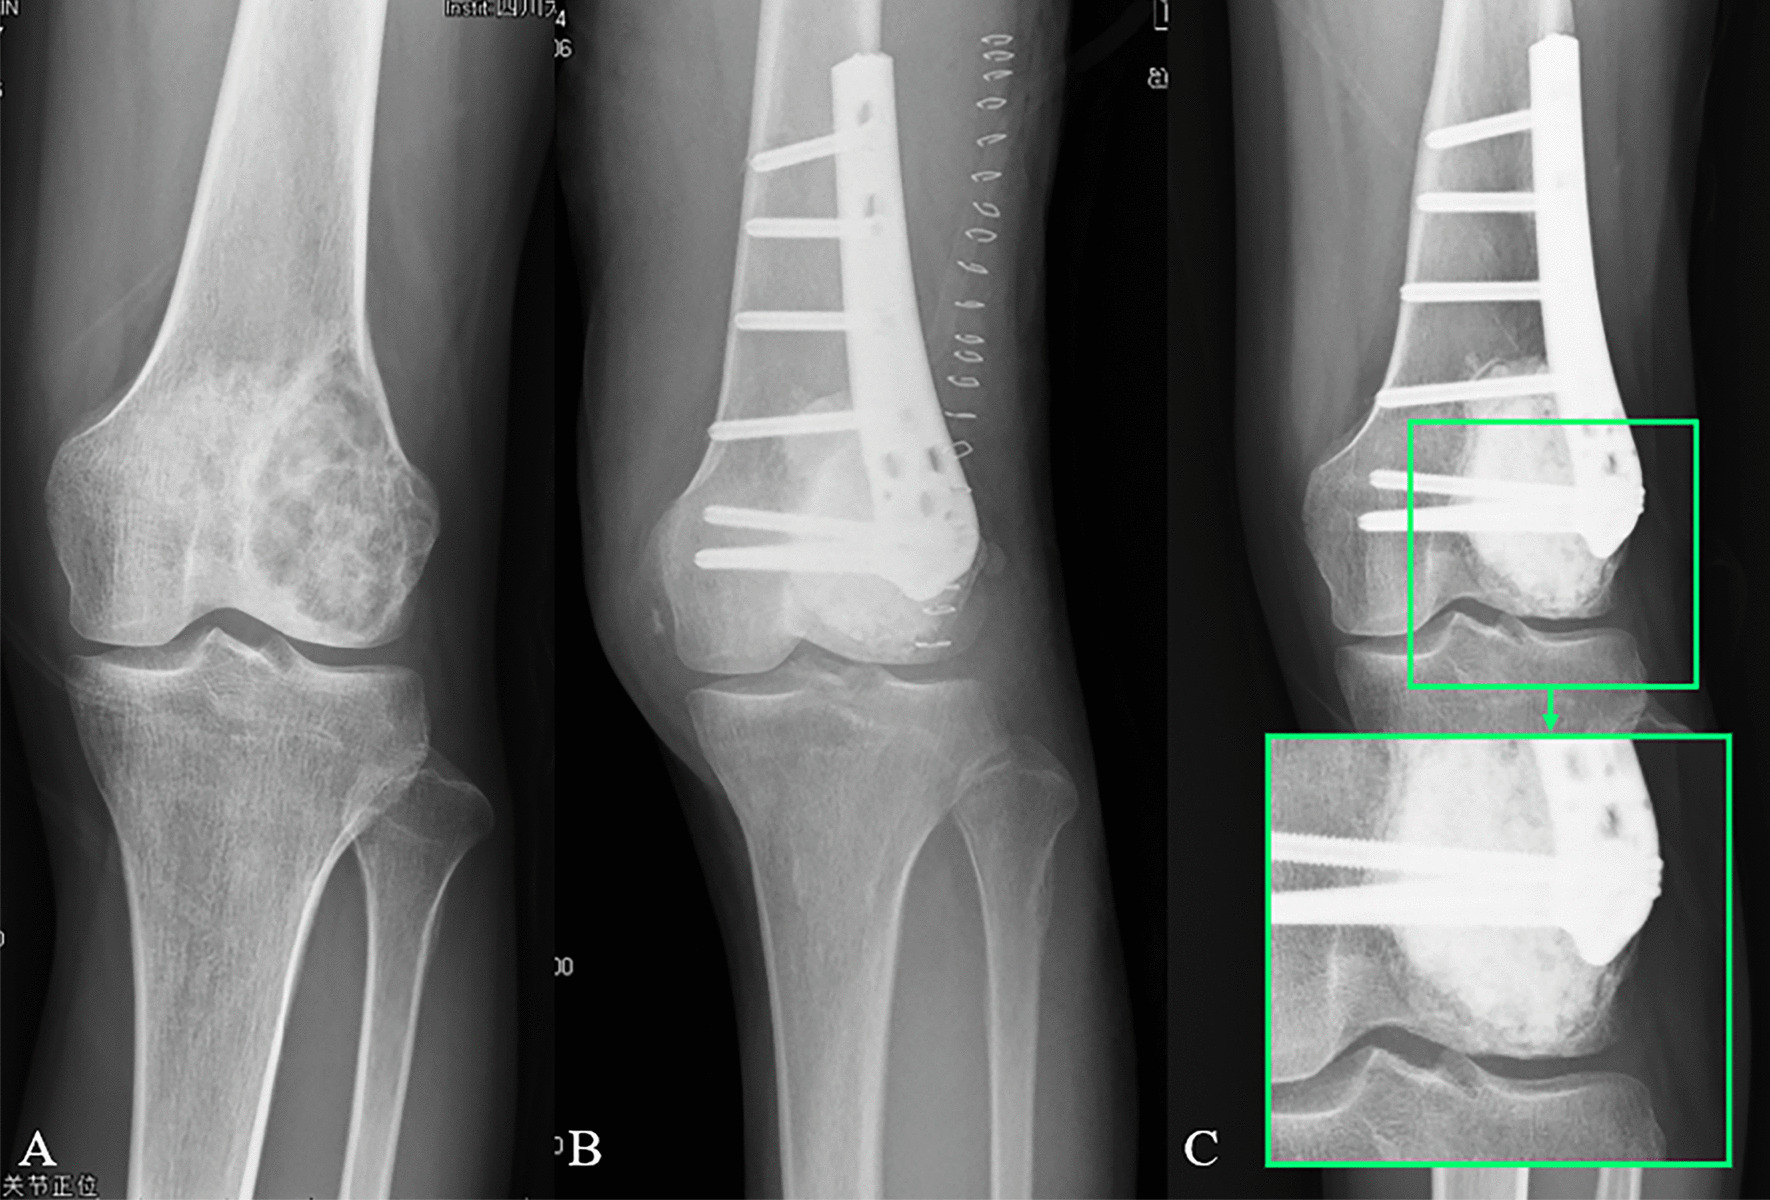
Preoperative and postoperative X-ray evaluations: A a AP view of a 29 years old male patient with GCTB. B Extended curettage, cement packing, subchondral bone grafting and plate-screws fixation were performed. C A sclerotic rim occurred (green box), and an interfacial gap between bone and cement can be observed
